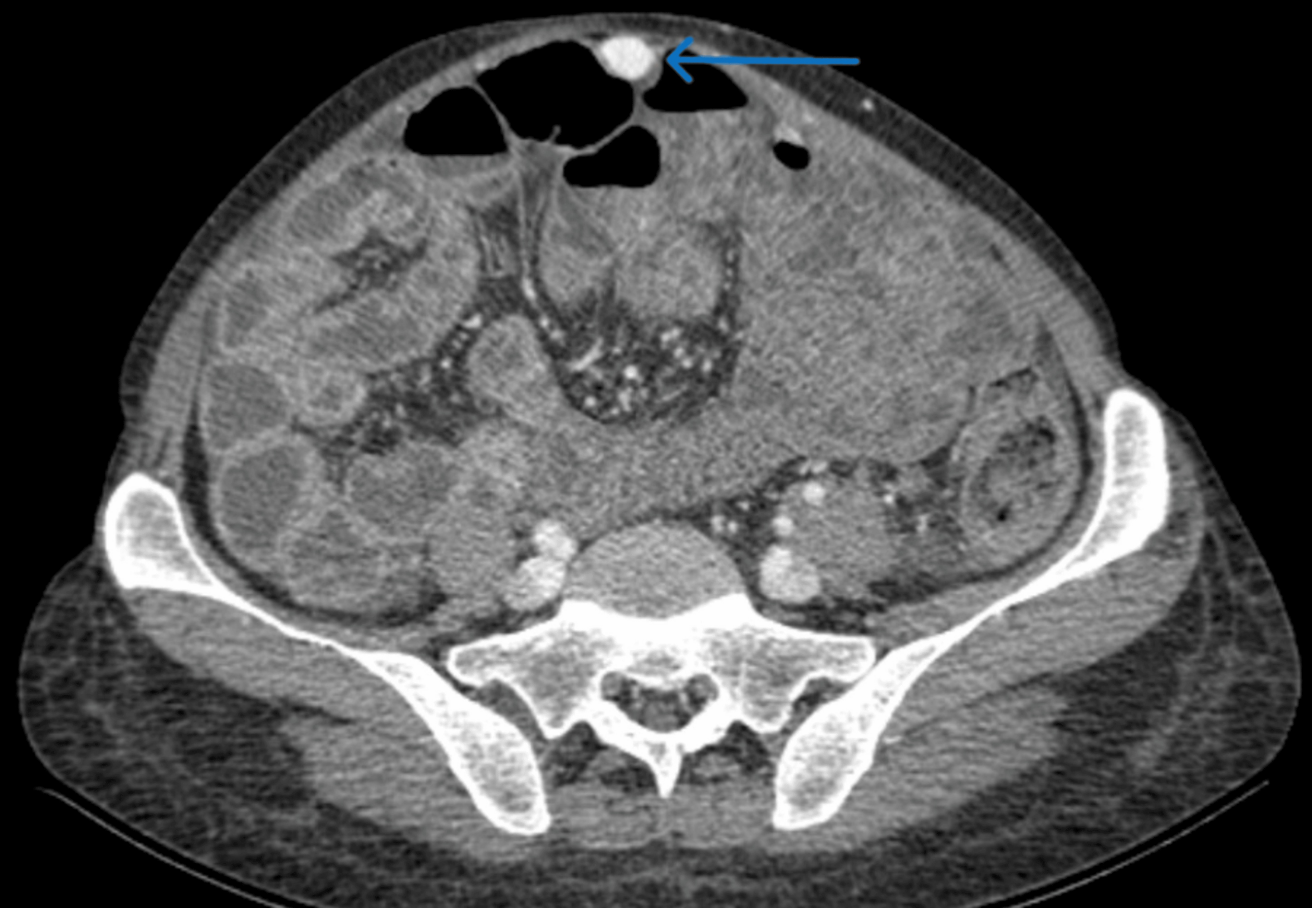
Portal-phase abdominal CT showing the superficial component of the shunt running within the anterior abdominal wall (blue arrow)
